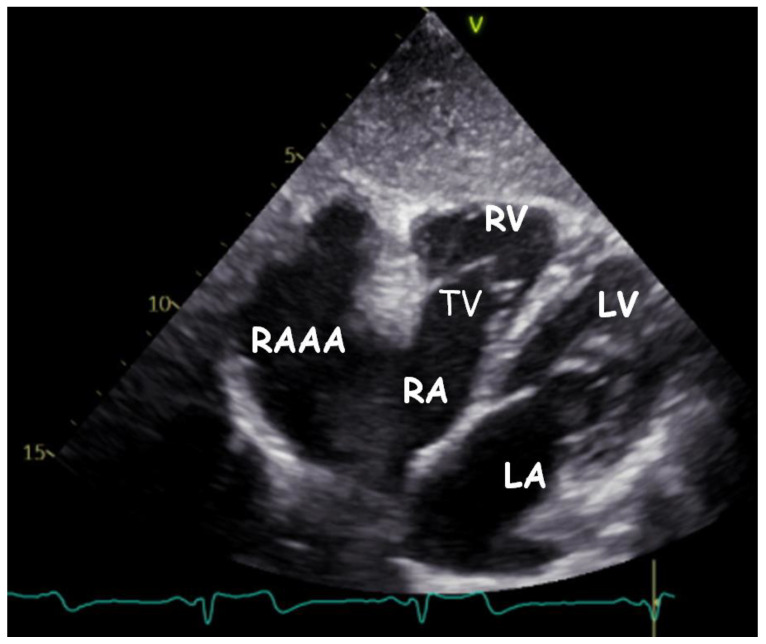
Transthoracic echocardiogram. Modified subcostal view showing the gigantic RAAA ten years later.
